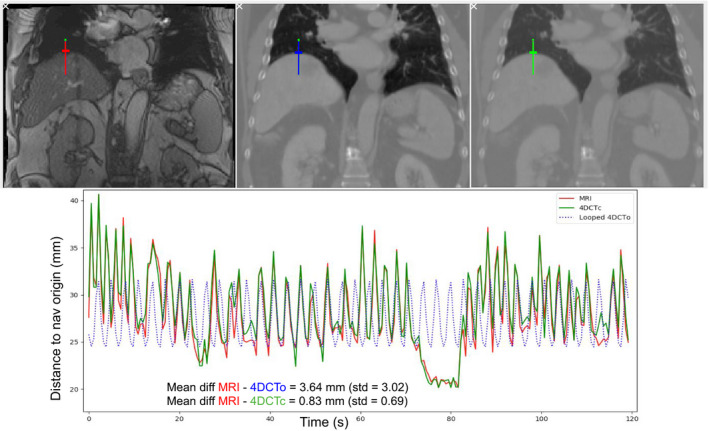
Example of motion comparison between the MRI sequence, the created 4DCTc and the looped 4DCTo and the resulting mean difference between motion signals.
